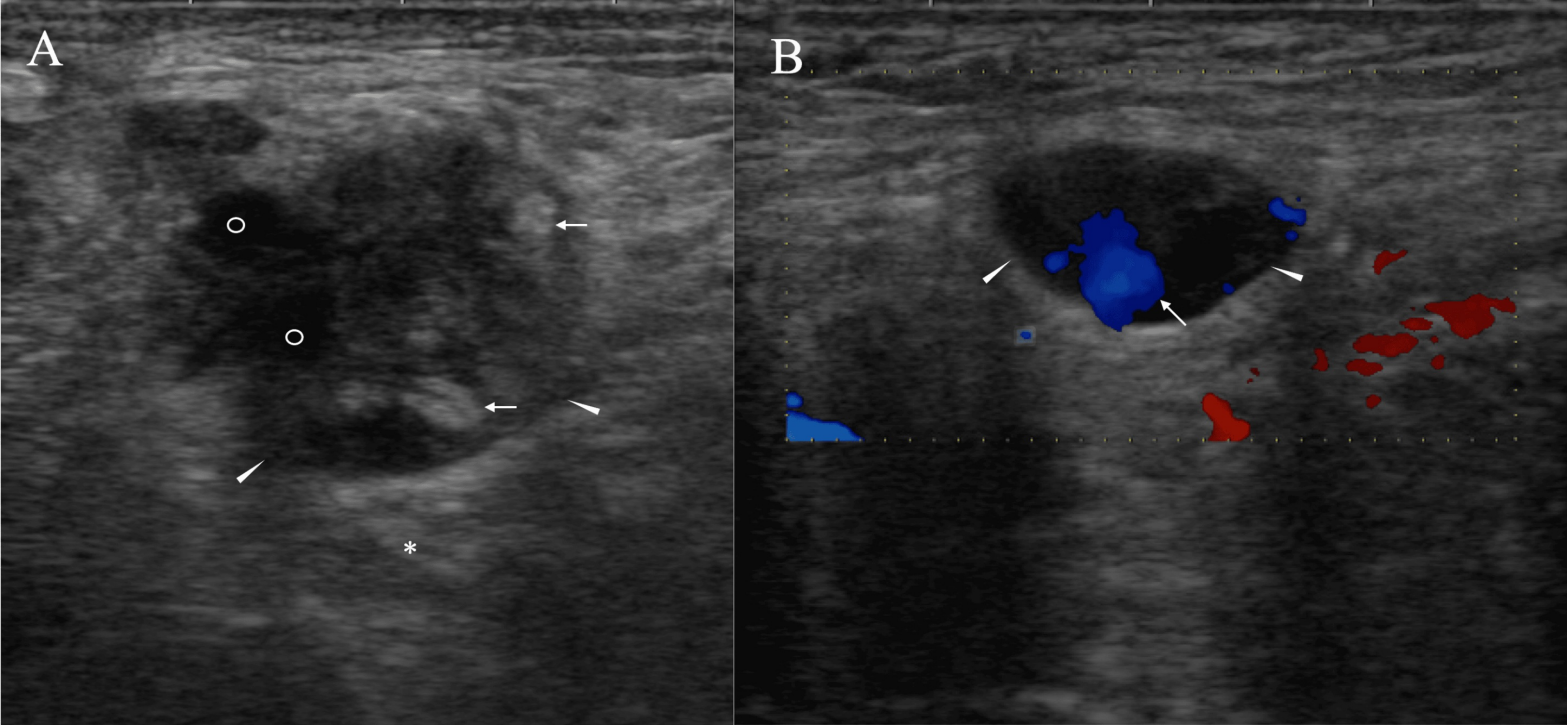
Ultrasound findings A) Ultrasound shows that one mass (arrowheads) has predominant internal high echoes, small very low-echo areas (open circles), and enhanced posterior echoes (asterisk). Very strong high-echo areas (arrows) appear to correspond to the focal adipocyte cluster areas. B) Ultrasound shows that another mass (arrowheads) has blood flow (arrow) into the mass.
